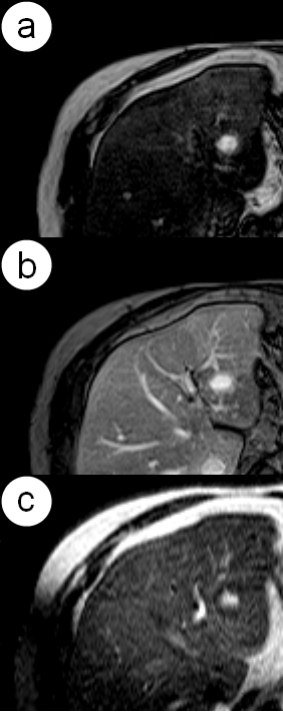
Hepatic hemangioma. MR study presents a homogeneous pattern of enhancement clearly visible in hepatic arterial (a) and portal venous (b) phases. T2-weighted MR image shows typical radiological finding of hemangioma - marked hyperintensity (c).
